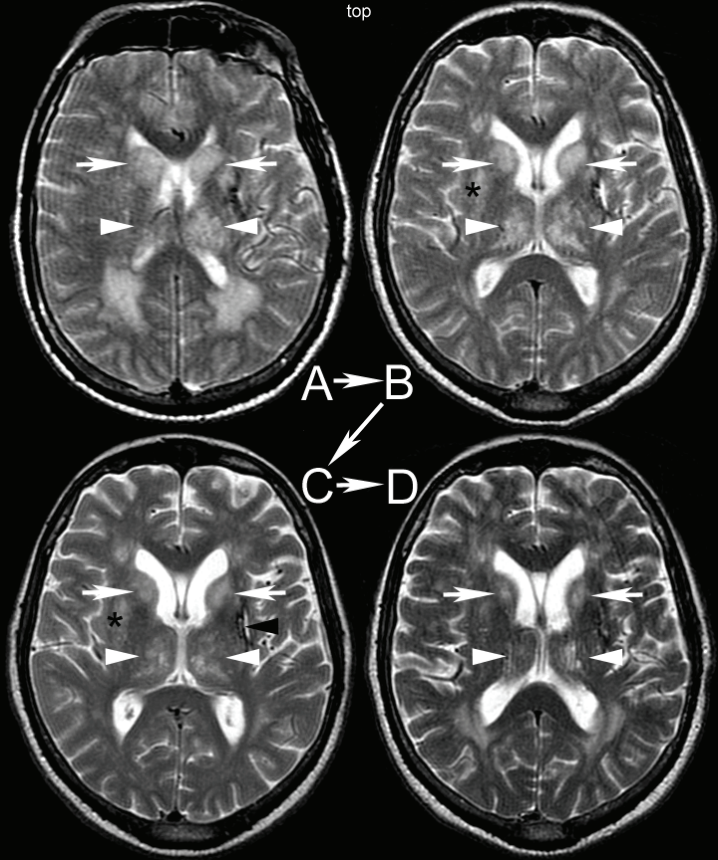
Serial brain magnetic resonance imaging (MRI) scans obtained during the course of the patient's illness. (A) Day 2: T2-weighted MRI scan demonstrates markedly enlarged caudate nuclei (arrows) and increased intensity in the thalami (arrowheads). (B) Eleven days after his initial presentation the abnormalities were resolving (arrows and arrowheads). Of note, the lenticular nucleus was involved (asterisk). (C) Three weeks and (D) two months after his initial presentation the abnormalities continued to improve, but had not resolved completely (arrows, arrowheads). Incidental hypertensive hemorrhage was present (black arrowhead in Figure 1C).
